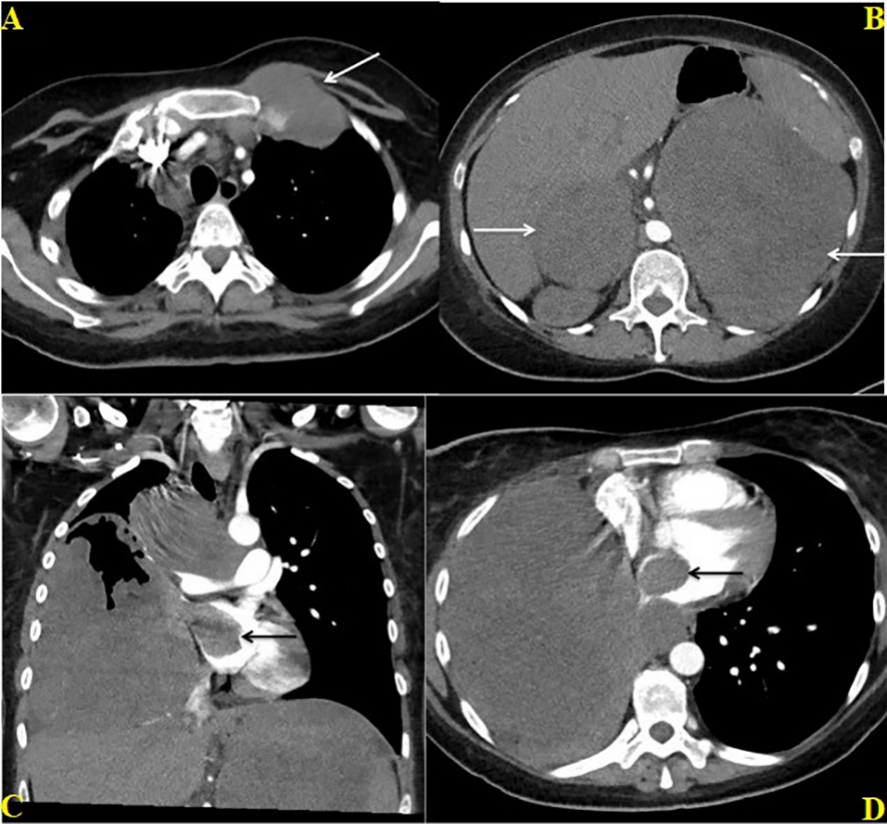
Contrast enhanced CT chest and abdomen dated June 27, 2022; Axial CT image through the chest (A) shows enlarged metastatic pre and paratracheal lymph nodes and a lobulated metastatic mass involving the left first rib (white arrow) at the costochondral junction. Axial CT image through the upper abdomen (B) shows large bilateral adrenal masses (white arrows). Coronal (C) and Axial (D) CT images through the chest shows a large hetereogenously enhancing mass in the right lung directly extending through the right superior pulmonary vein into the right atrium (black arrow) and conglomerate paratracheal lymphadenopathy (C).
